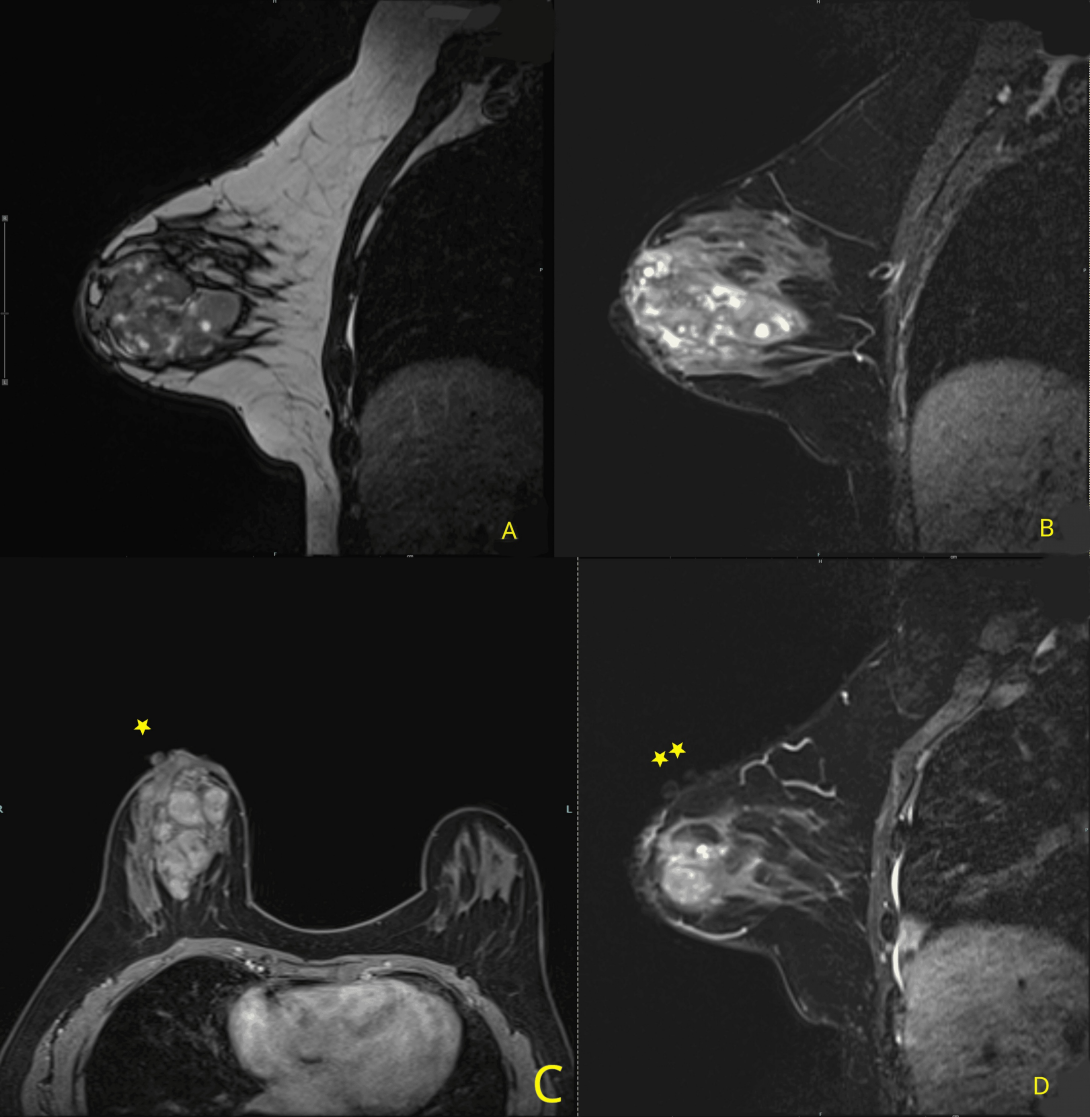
MRI findings (A) Sagittal T1-weighted image and (B) Sagittal T2-weighted image show that the right breast parenchyma presents a heterogeneous nodule with cystic areas inside. (C) Axial T1-weighted fat sat C+ (gadolinium) shows no enhancement, neither the dermal lesions (yellow stars) nor the parenchymal nodules, post-contrast administration. (D) Sagittal T2-weighted image shows the dermal lesions (yellow stars) of the same intensity, following the path and contour of the skin MRI: magnetic resonance imaging
